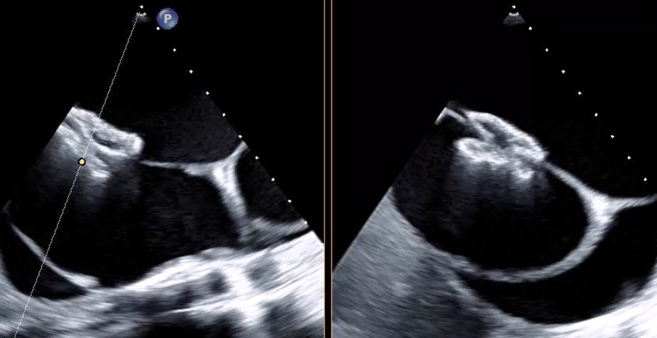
Final Result of Emergency Iatrogenic ASD Closure Transesophageal echocardiography after device release. Still image demonstrating the 18-mm Occlutech Figulla Flex II ASD occluder in its final position. The thinned, overstretched interatrial septum is securely sandwiched between the disks. This was achieved through a stationary deployment technique (Video 4) to avoid further mechanical tearing. The precise alignment ensures complete shunt elimination while preserving the integrity of the friable tissue. ASD = atrial septal defect.
